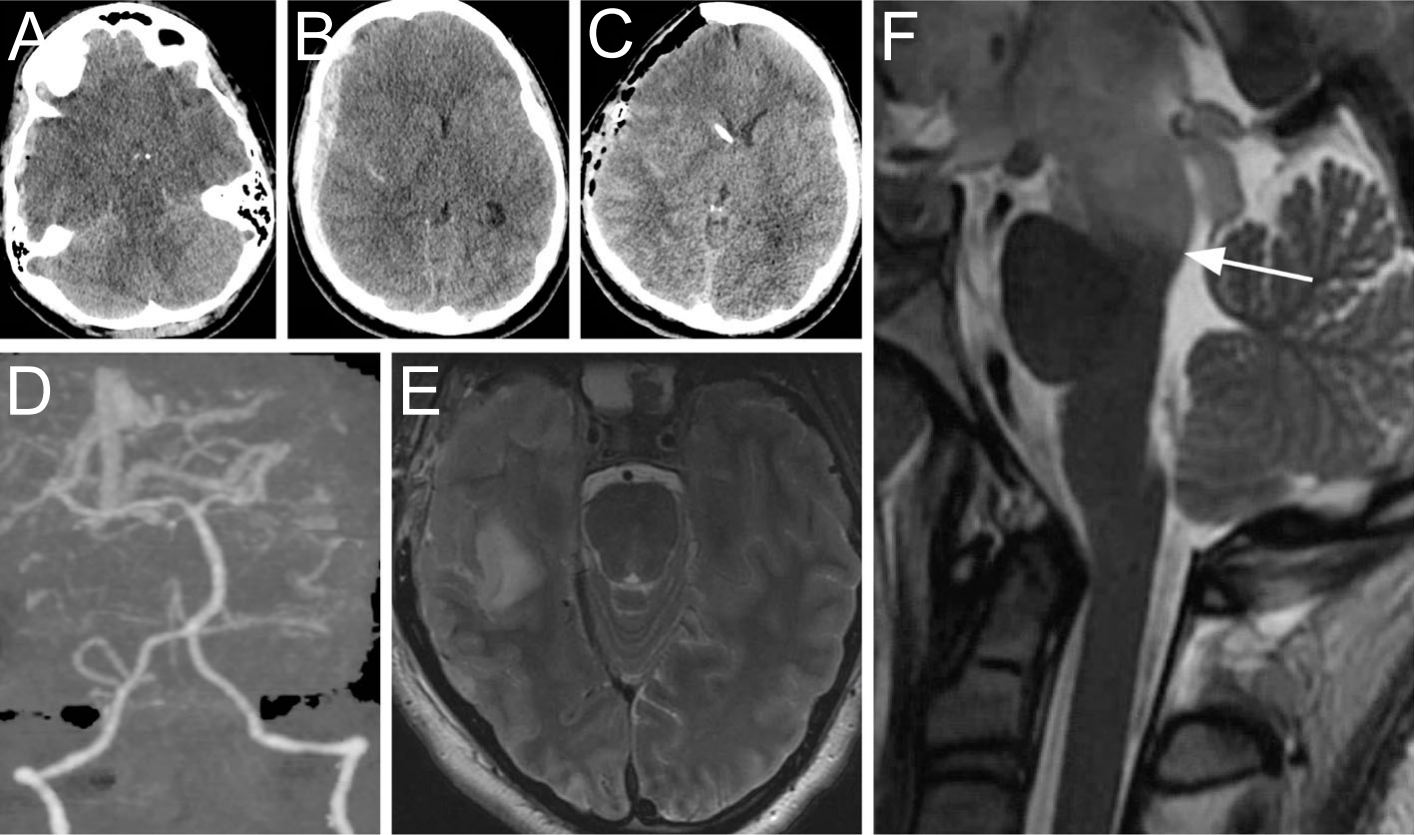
a, b Axial noncontrast head computed tomography (CT) scan on presentation showing effacement of the basal cisterns (a) and a right convexity acute subdural hematoma causing midline shift (b). c Axial CT scan immediately following right hemicraniectomy and ventriculostomy placement showing relief of mass effect and evacuation of hematoma. d Three-dimensional CT angiography reconstruction showing patent posterior arterial and deep venous circulation. e, f T2-weighted magnetic resonance axial (e) and sagittal (f) images of the cervical spine 18 h postoperatively. The flow void of the basilar artery is visualized at the mid-pons, and a right temporal contusion is evident (e). The sharp transition at the pontomesencephalic junction (arrow) highlights hyperattenuation of the midbrain and diencephalon (f), with sparing of the remainder of the brainstem
